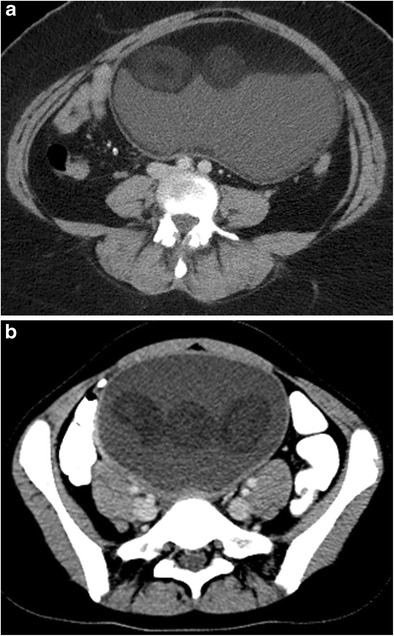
Axial CT image of an 18-year-old female with mature cystic teratoma (MCT) (a) shows two rounded floating balls in the interface of the fat-fluid level. The bigger one has a low density core and a low density outer shell. In another case with MCT (b), floating balls have relatively high density cores
